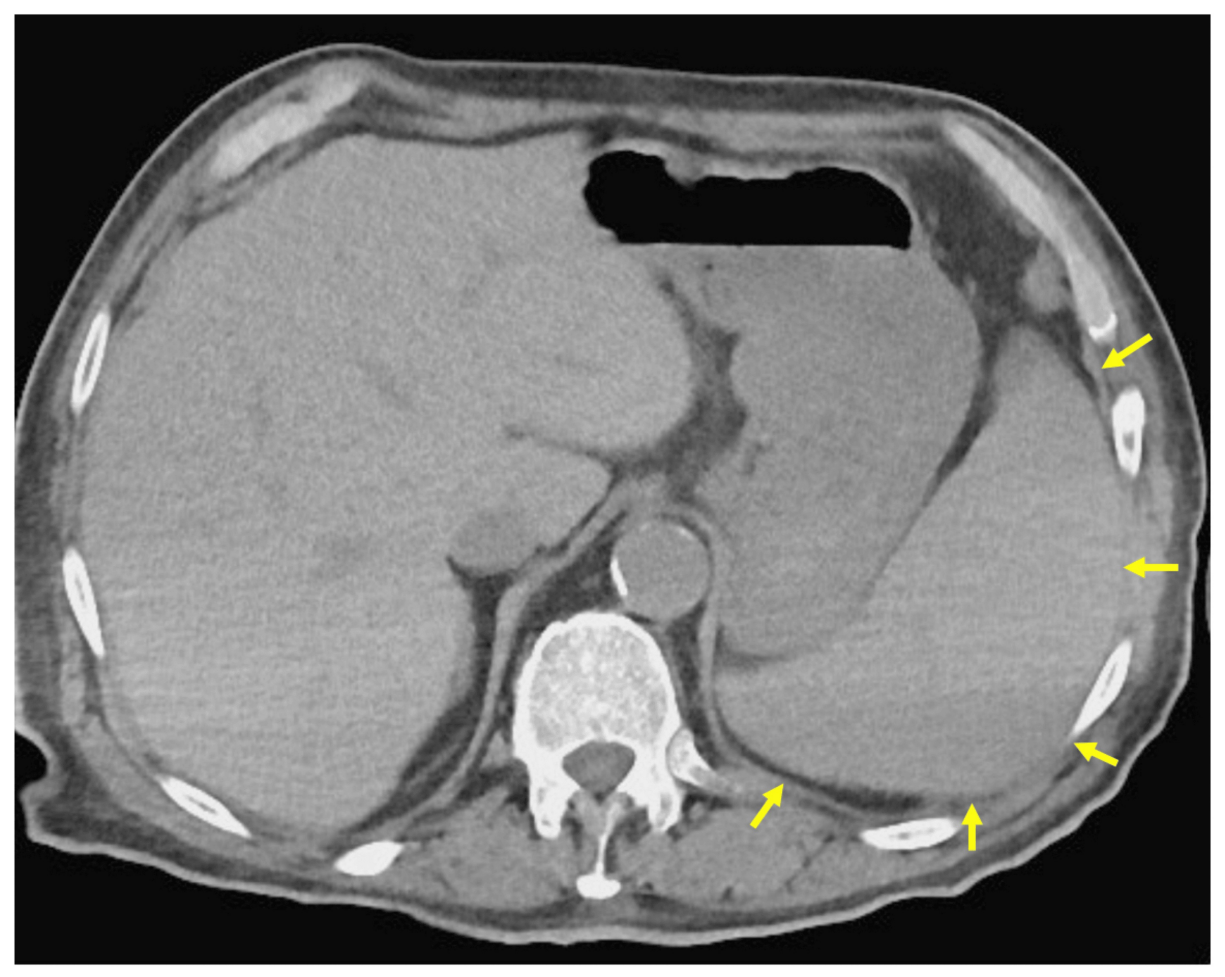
Abdominal computed tomography An axial abdominal computed tomography image reveals splenomegaly (arrow)
